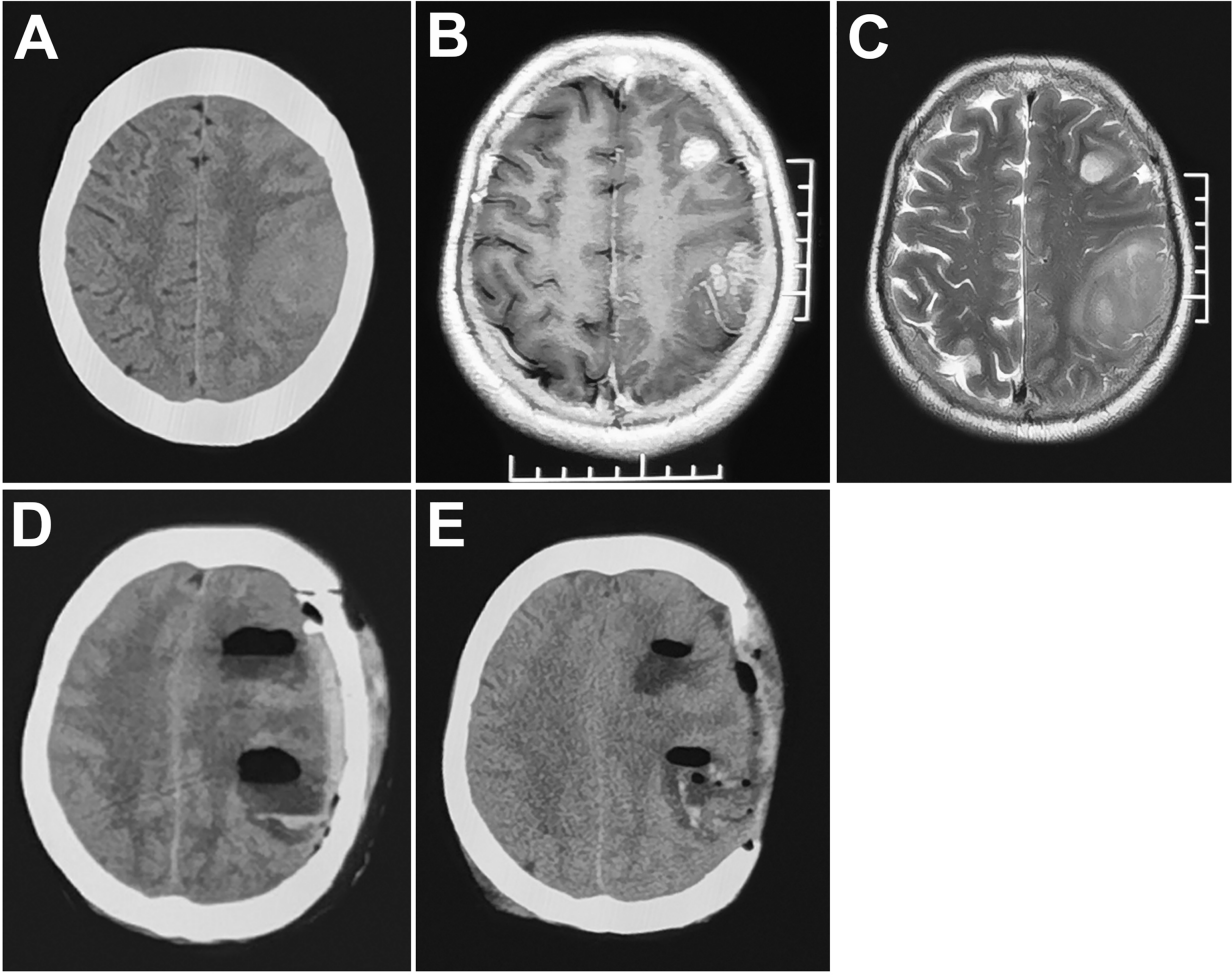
MRI (A–C) multiple gliomas in the left frontoparietal lobe. CT (D) obvious midline shift and cerebral venous infarction (cortical veins were injured during the operation) 72 hours postoperatively. (E) decompressive craniectomy.
